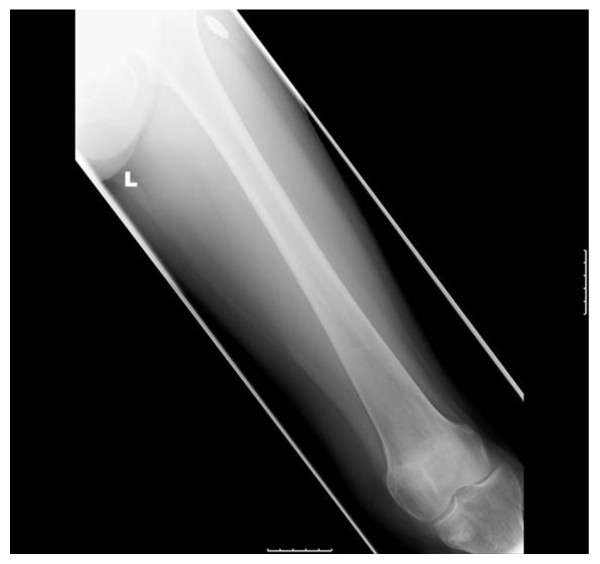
Anteroposterior radiographs of the left femur six months after diagnosis with resolution of symptoms and healed fractures.
